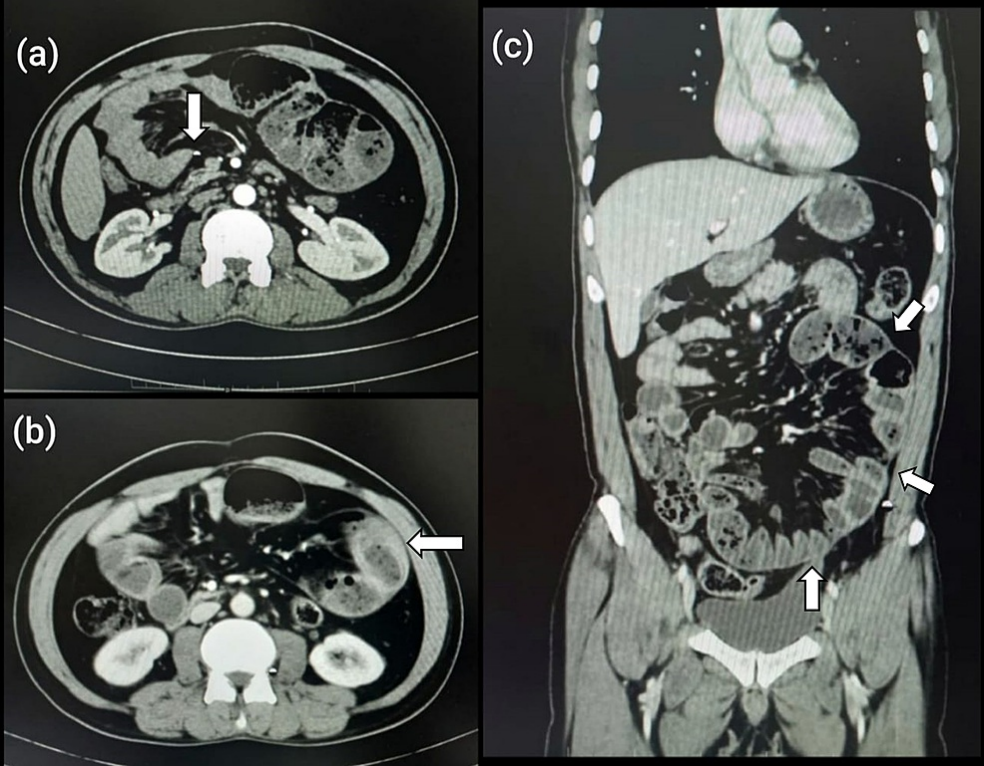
CECT abdomen images (a) Axial section with the arrow showing obstruction in the jejunum. (b) Axial section with the arrow showing venous congestion around dilated loops. (c) Coronal section with arrows showing cocoon outline with dilated bowel loops. CECT - Contrast-enhanced computed tomography
